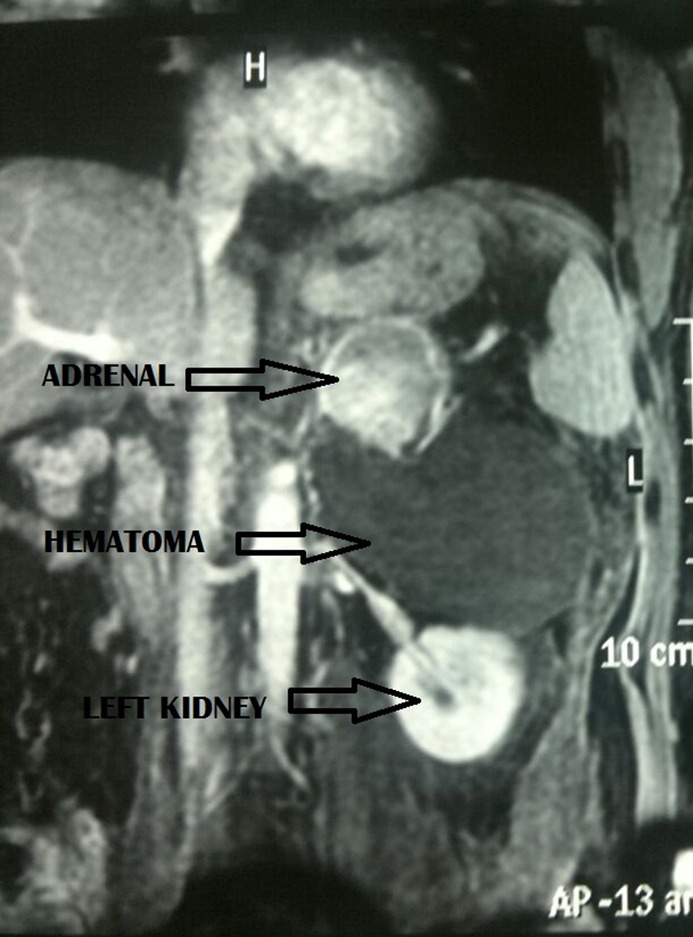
MRI showing left suprarenal mass with large hemorrhagic component, suggestive of spontaneous hemorrhage in an adrenal mass, likely representing pheochromocytoma.
